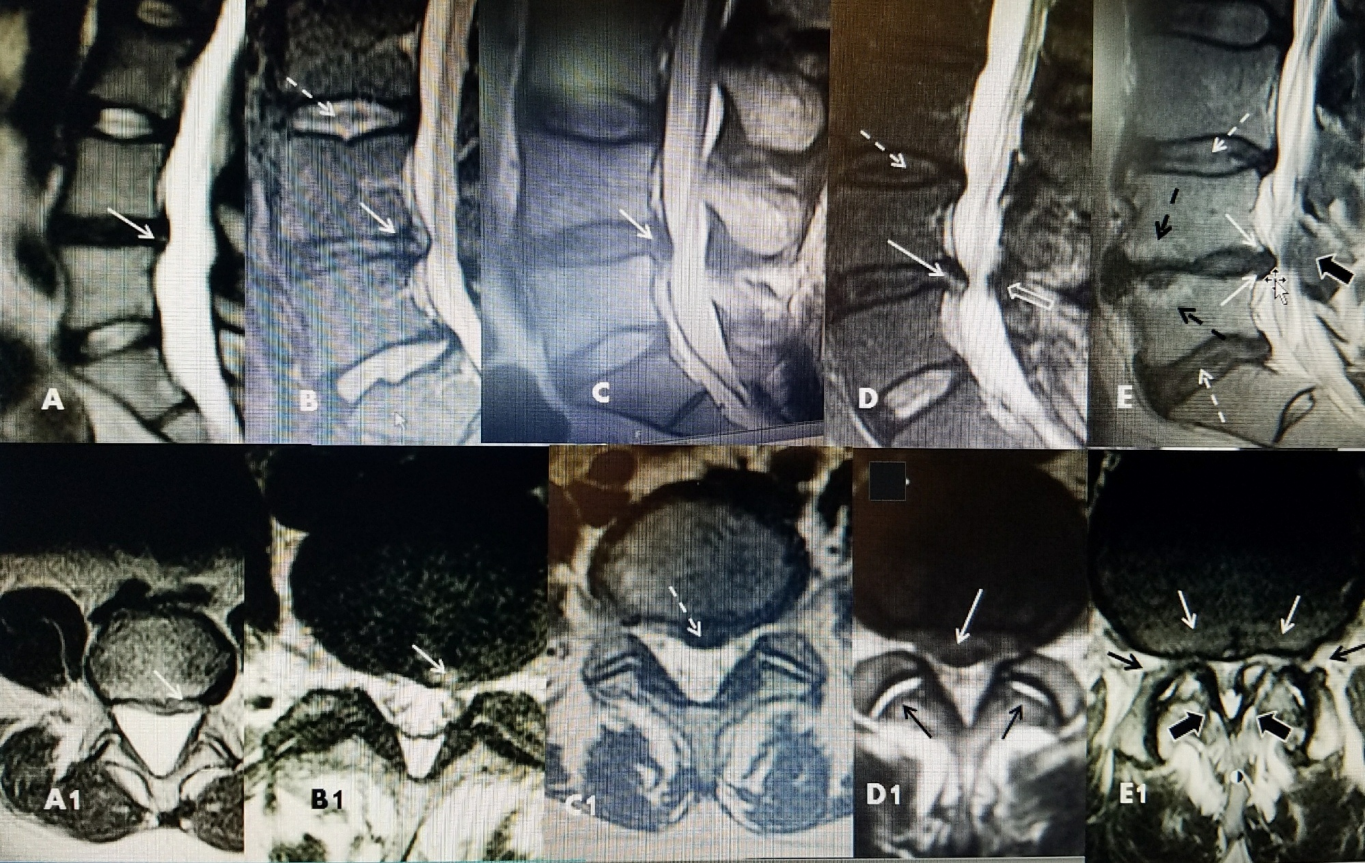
Range of sizes of L4-5 herniated discs A/A1: Sagittal and axial magnetic resonance image (MRI) of small L4-5 disc with 2 mm herniation but marked T2 signal change indicating disc dehydration and dessication. White arrow shows midline herniation. B/B1: Sagittal and axial MRI showing more distinct annular tear and sub-annular slightly superior herniation (solid white arrow). There is some slight narrowing of the vertical height of the disc space. There is a dessicated line in the center of the disc space above indicative of early Pfirrmann changes (dotted white arrow). C/C1: Sagittal and axial MRI showing midline 3-4 mm herniation with more distinct part inferiorly into the epidural space under the posterior longitudinal ligament. D/D1: Larger 6 mm herniation that is still contained but in the anterior epidural space (solid white arrow). There is minimal but distinct posterior ligamentum hypertrophy. The combination is causing localized canal stenosis (open white arrow). There is signal change in the disc above indicative of early dessication (dotted white arrow) with a small annular bulge. On the axial film there is high intensity fluid in both facet joints indicative of joint inflammation (black arrows). E/E1: MRI showing marked disc space narrowing at L4-5 with Modic changes in the anterior half of the both endplates (dotted black arrow). There is more distinct annular fibrotic thickening ( 2 solid white arrows) causing ventral compression (dotted cross and small white arrow) in the anterior epidural space. Posteriorly there is narrowing secondary to hypertrophied ligamentum. The facet joint is better seen on axial view in E1 (black arrow with white border) and there is a large calcified portion of the facet indenting the medial canal. The combination causes marked canal stenosis. On axial view there is bilateral neural foramina stenosis (solid black arrows).
